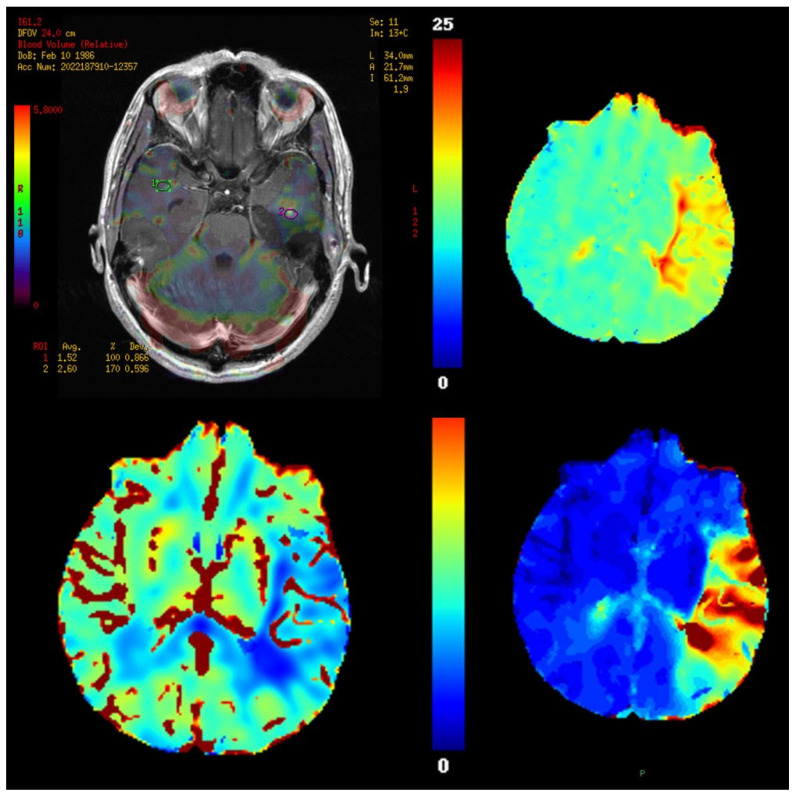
DSC-MRI perfusion parametric maps. (Top-left): relative cerebral blood volume (rCBV) overlaid on the structural reference, with ROIs placed in the lesion and contralateral white matter for ratio calculation. (Top-right): relative cerebral blood flow (rCBF) map. (Bottom-left): mean transit time (MTT) map. (Bottom-right): time-to-peak/bolus arrival delay map. Warm colors indicate higher rCBV/rCBF or prolonged transit/arrival times, whereas cool colors indicate lower values/shorter times. Maps were derived from a single-bolus T2*-weighted EPI DSC acquisition using AIF-based deconvolution (with leakage correction) and are shown with arbitrary but consistent color scales.
